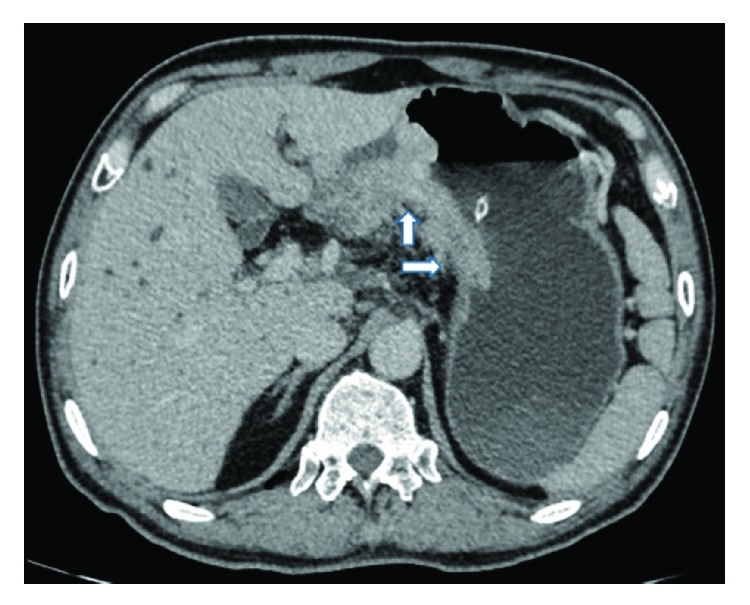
CT image obtained in a 56-year-old man. The serosal surface is rough around the lesser curvature of the stomach.
